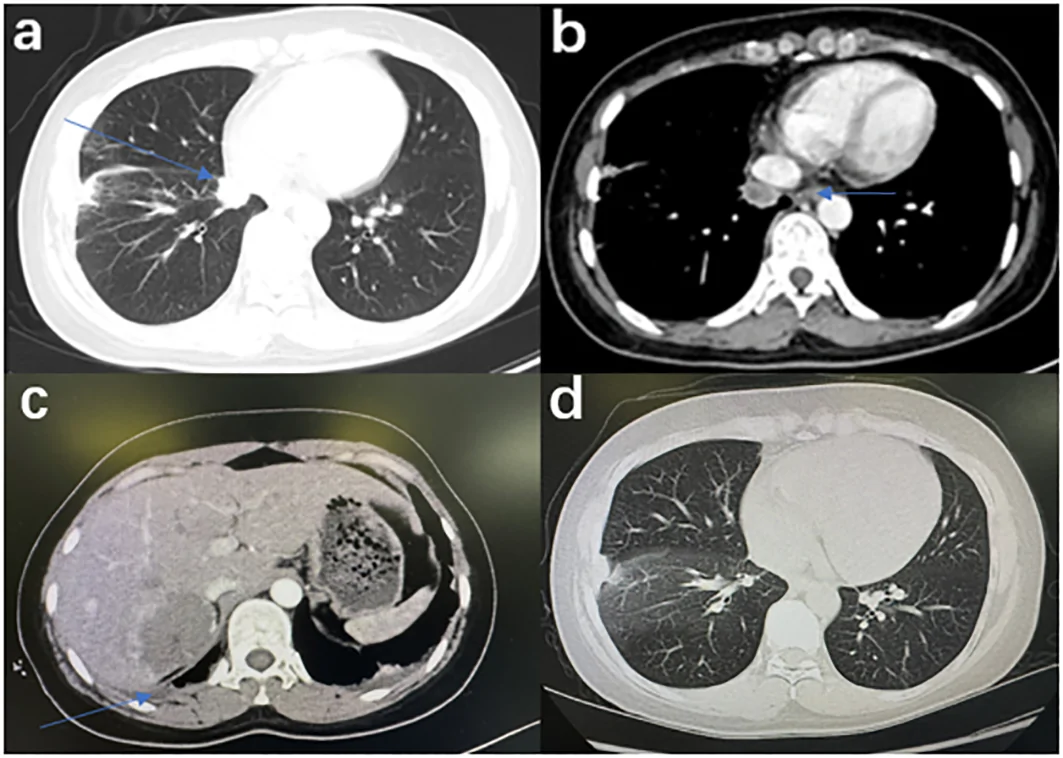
Initial and follow-up chest CT findings. (a) Plain CT scan (THK: 2 mm) showing a soft tissue nodule (arrow) measuring approximately 2.0 × 1.7 cm near the hilum of the right lower lung. (b) Contrast-enhanced CT scan (THK: 5 mm) demonstrating enlargement of right hilar and mediastinal lymph nodes (arrowheads). (c) Contrast-enhanced CT showing bilateral pleural effusion (arrows). (d) Follow-up contrast-enhanced CT after six months of alectinib therapy demonstrating partial response.
